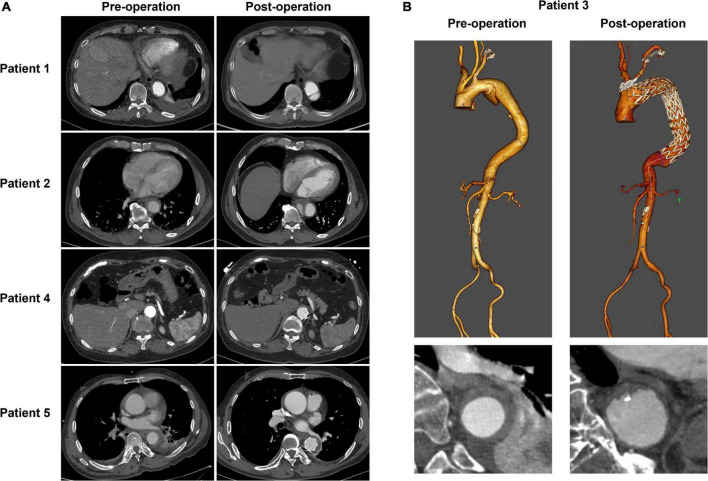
The CTA image changes of five TEVAR patients during follow-up. (A) The cross-section images of patient1, 2, 4, and 5. (B) 3D reconstruction of CTA image of patient 3.
